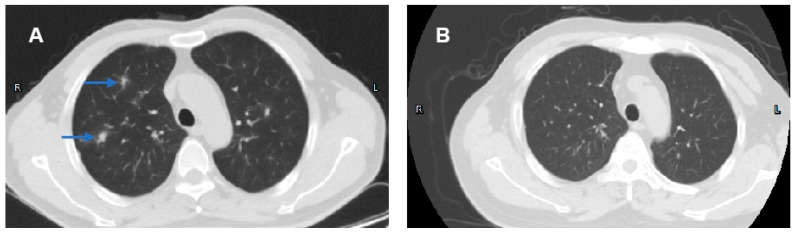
CT scan before (A) and after (B) the steroids therapy. (A) CT scan before the treatment (June 2022). The results displayed interstitial inflammation and multiple ground-glass nodules (blue arrows) in lungs. (B) CT scan after the treatment (December 2022). Interstitial inflammation and multiple ground-glass nodules decreased significantly in lungs after steroid therapy.
